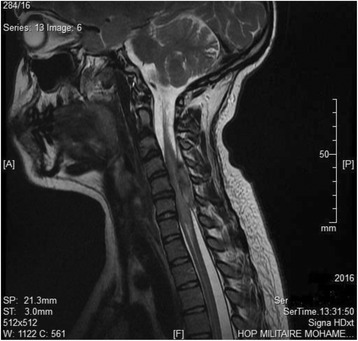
T2-weighted sagittal magnetic resonance image of the cervical spine showing an epidural hematoma located dorsal to the cord extending from C3 to C6 levels, causing obliteration of the spinal subarachnoid space and pushing the cord anteriorly against the vertebral bodies
